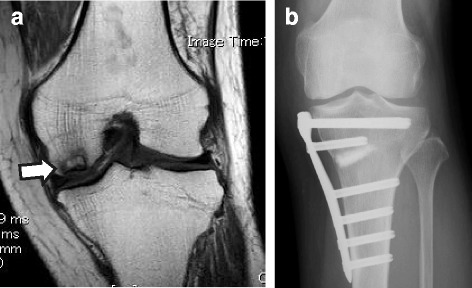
Frontal images of the knee joint in a 67-year-old woman who underwent opening wedge high tibial osteotomy for osteonecrosis of the knee. a The lesion area visualized by preoperative magnetic resonance imaging is shown with an *arrow*. b Postoperative radiograph
